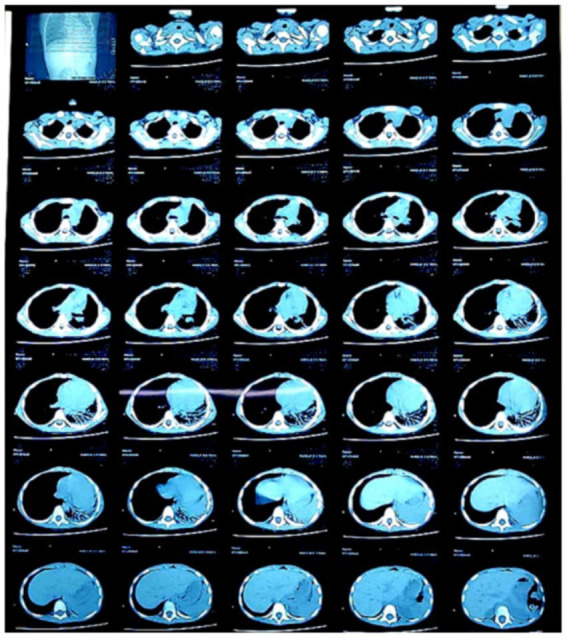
Chest high resolution computed tomography conducted a month before presenting to our hospital—showing a patch of consolidation with internal air bronchograms and sub-segmental collapse in the left lower lobe with mild ipsilateral cardio-mediastinal shift.
